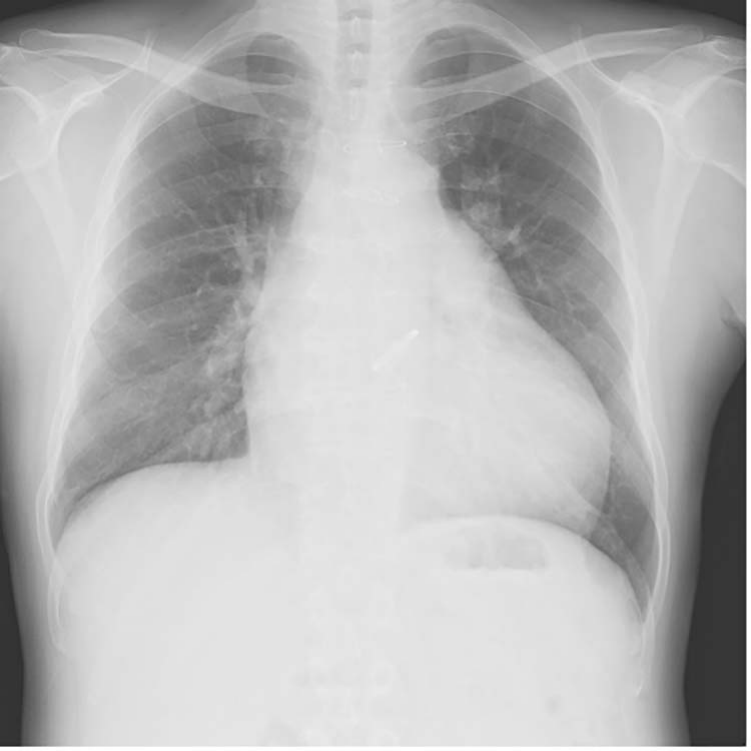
Plain chest radiograph of the first outpatient visit after discharge from the hospital.
